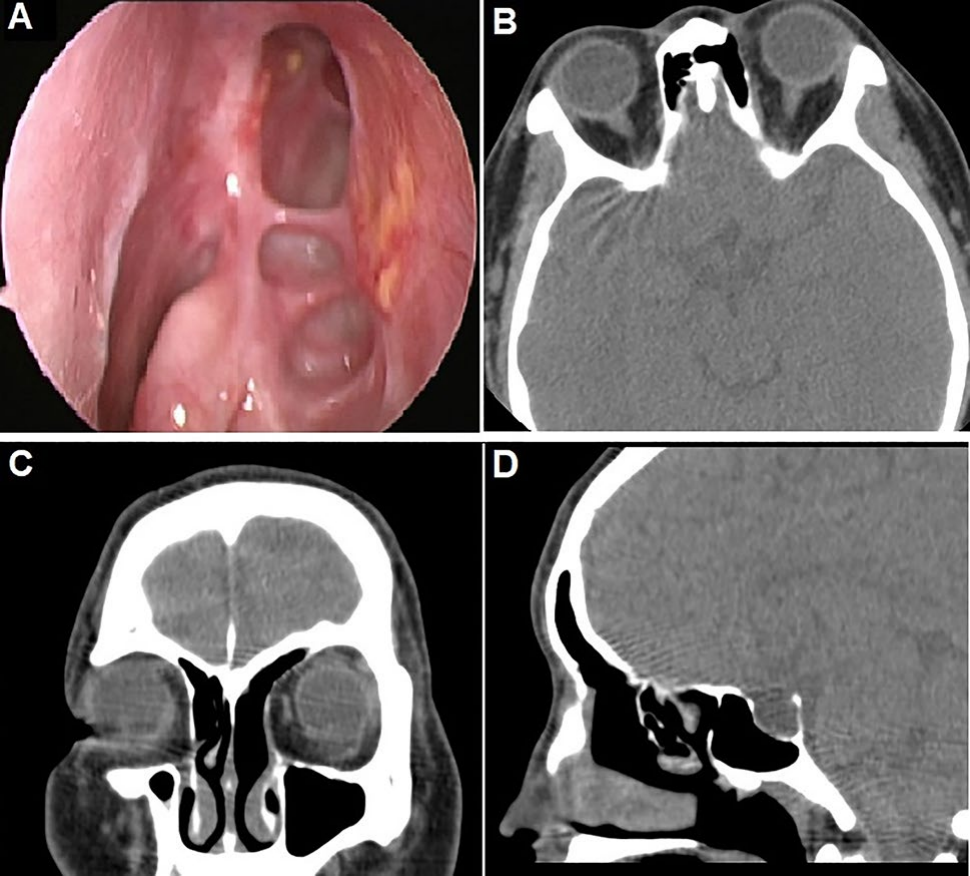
(A) Endoscopic views, (B) Axial, (C) coronal and (D) sagittal CT images showing the neo-ostium at 12 months after surgery
